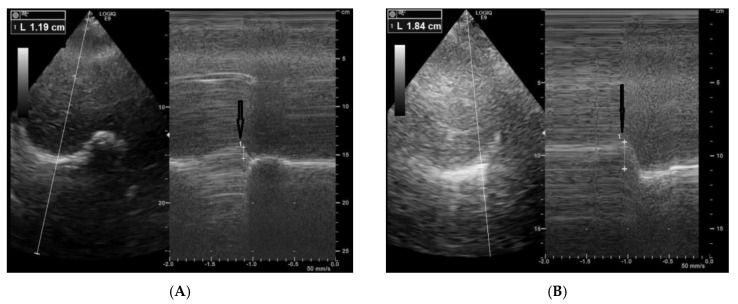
(A) (−11 mm) and (B) (−18 mm) hemi diaphragm paradoxical displacement using M mode ultrasound during a sniff maneuver in patient with LOPD.
